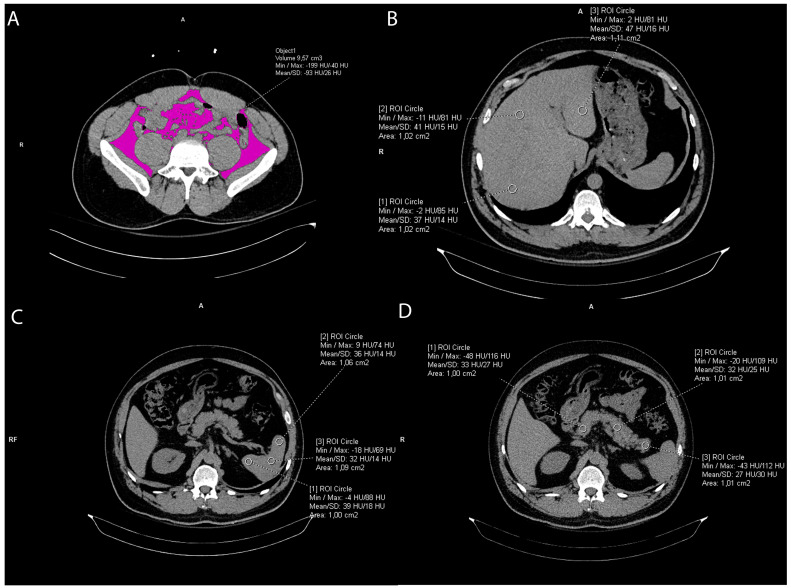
Representative axial CT images showing visceral fat volume calculation (A), liver attenuation measurement (B), spleen attenuation measurement (C), and pancreas attenuation measurement (D).
